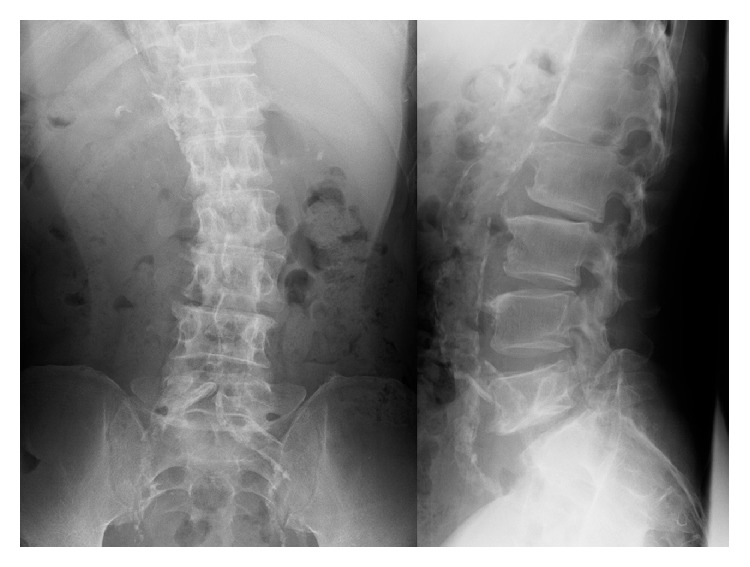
Plain radiographs showing a degenerative lumbar scoliosis and Meyerding grade I L5-S1 isthmic spondylolisthesis 2 years before surgery.
